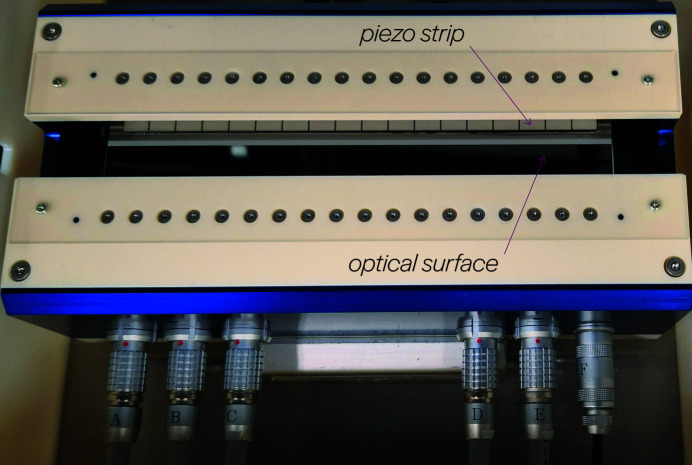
Piezo-bimorph deformable X-ray mirror. The adaptive X-ray mirror (JTEC Corporation) that was studied and characterized in our experiments. A segmented piezo strip enables spatially localized deformations of the optical surface.
